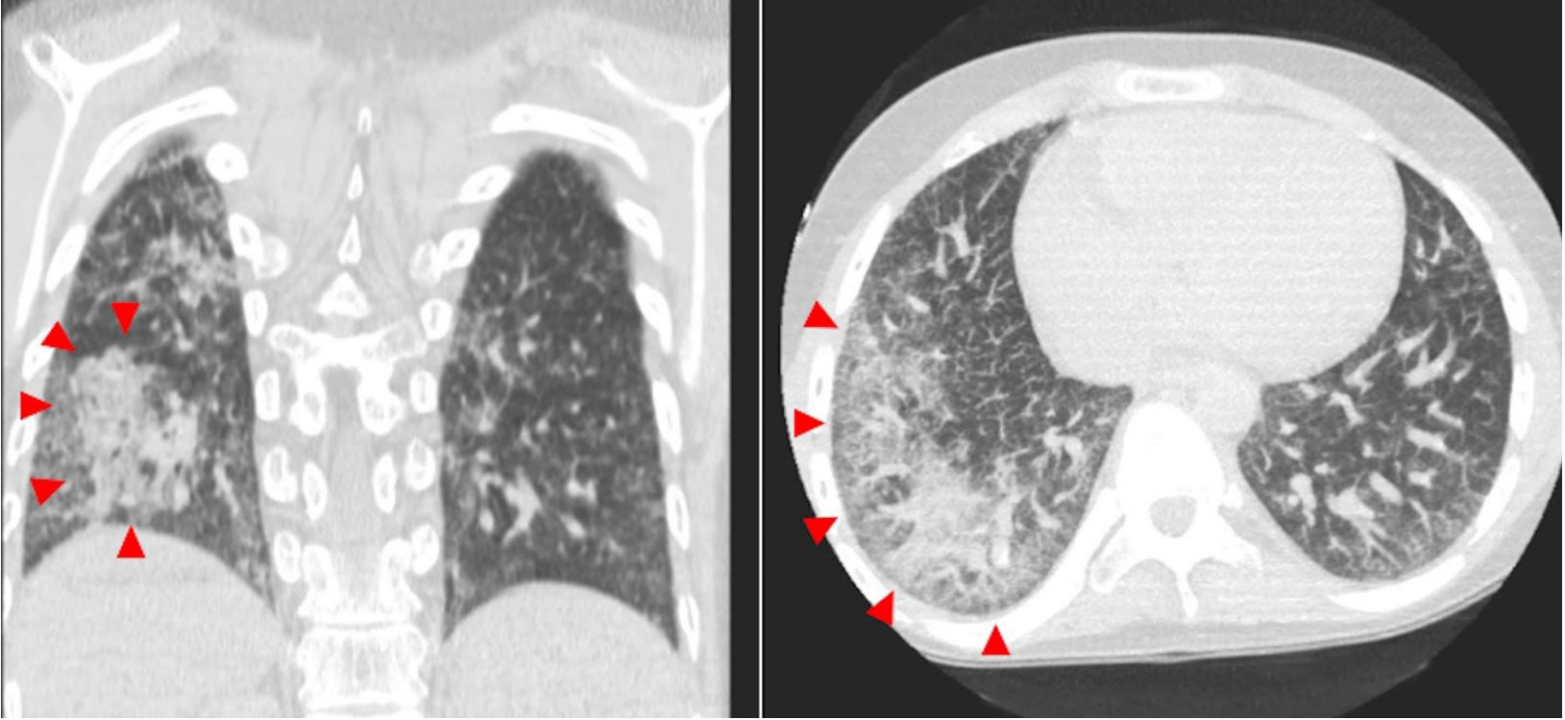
CT scan of the chest showing extensive reticulation, interlobular septal thickening, and patchy confluent areas of peripheral airspace consolidation on the right lung (red arrowheads).
